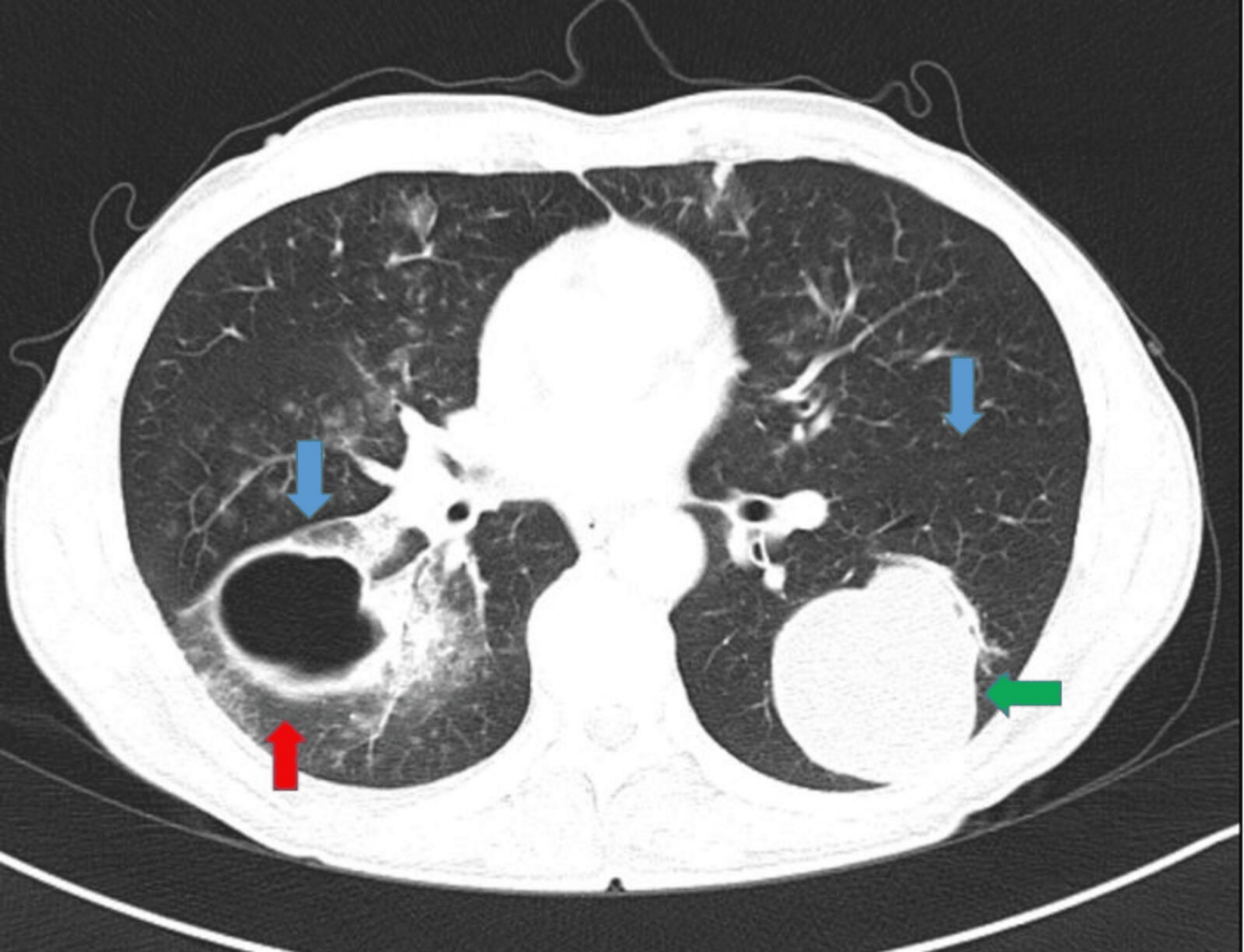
Thorax computed tomography parankim window shows bilateral hydatid cysts Major fissures are marked with blue arrows. The red arrow shows a perforated cyst related to a fissure. The green arrow shows an intact cysts not related to a fissure
